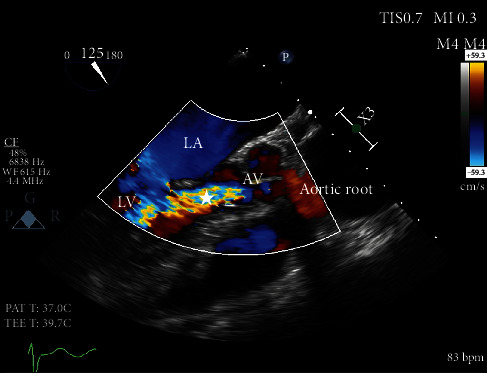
Mid epigastric long axis view. In Figure 3, aortic valve doming is seen during midsystole, suggesting asymmetry and restriction. In Figure 4, taken with doppler ultrasonography during middiastole, a “mosaic pattern” (star) is evident in the left ventricular area, suggesting aortic regurgitant flow.
